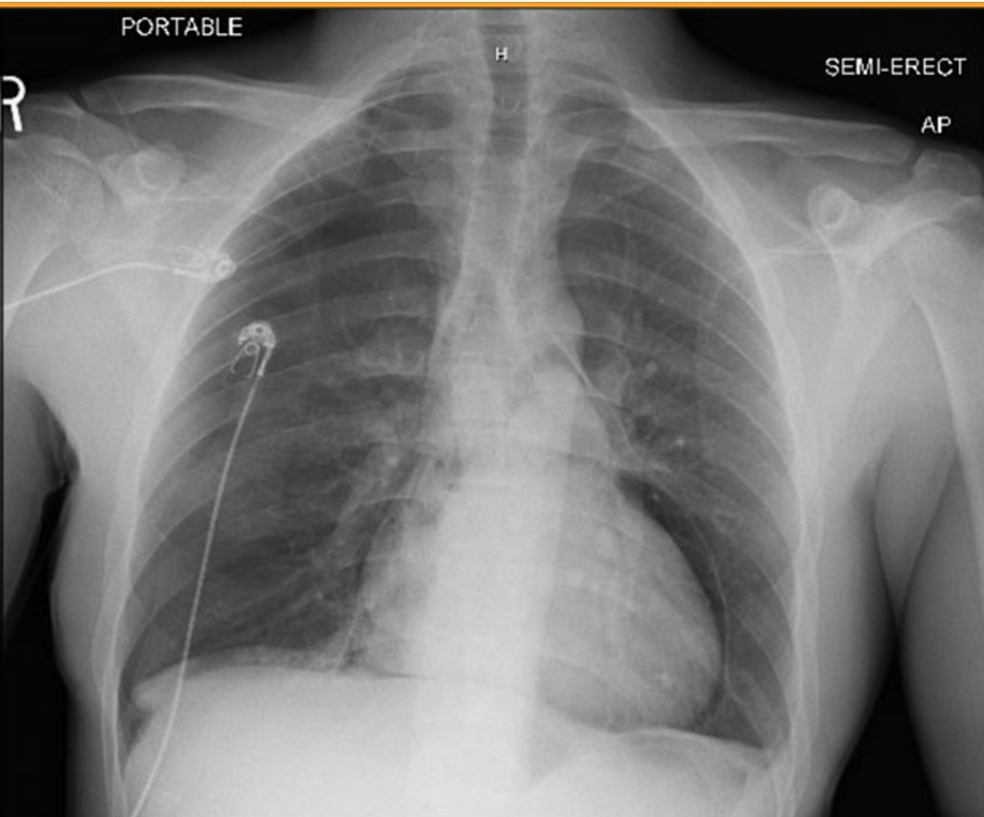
Initial chest x-ray on presentation to the emergency department
